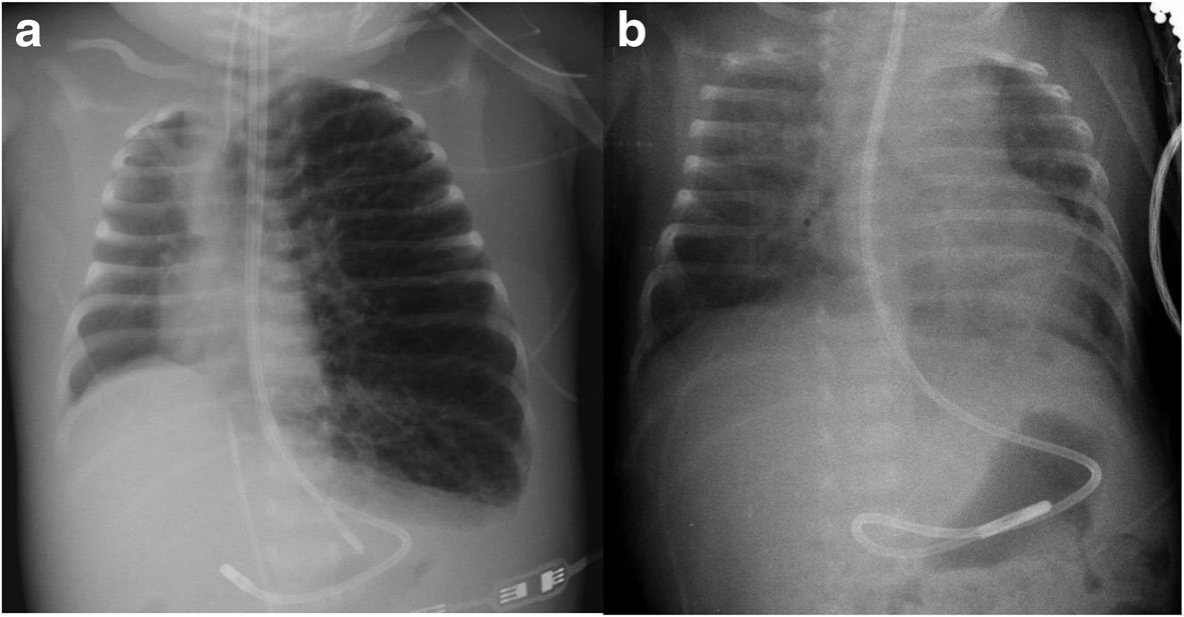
Chest X-Rays showing left lung hyperinflation with cystic images on the day of intubation a and a marked improvement after 5 days of ECMO support b
